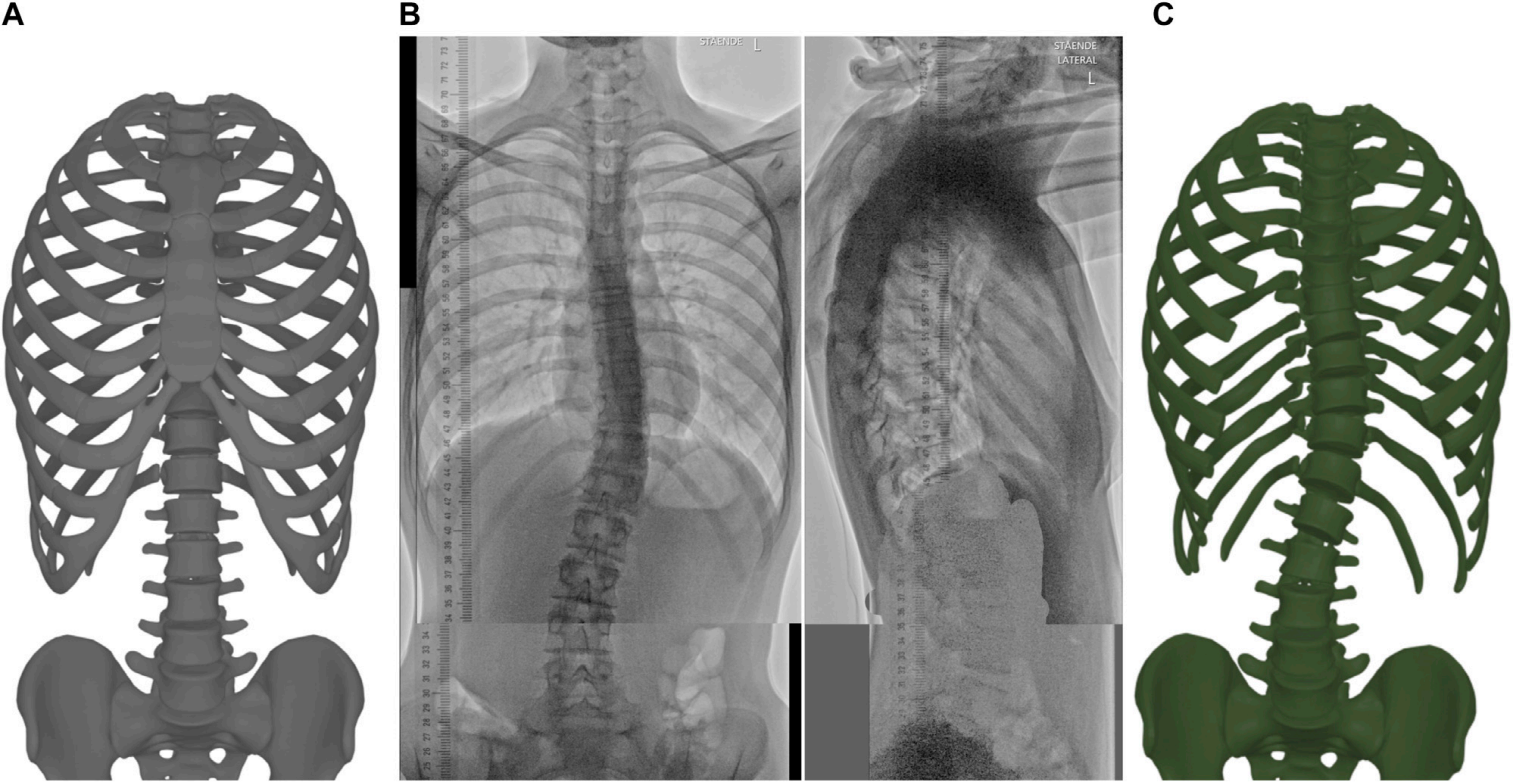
The proposed method takes as input a template model of the torso skeleton (A) and biplanar low-dose radiographs (B), and outputs the morphed torso skeleton (C). Here we hide the sternum and the costal cartilages to clearly show the spine.
